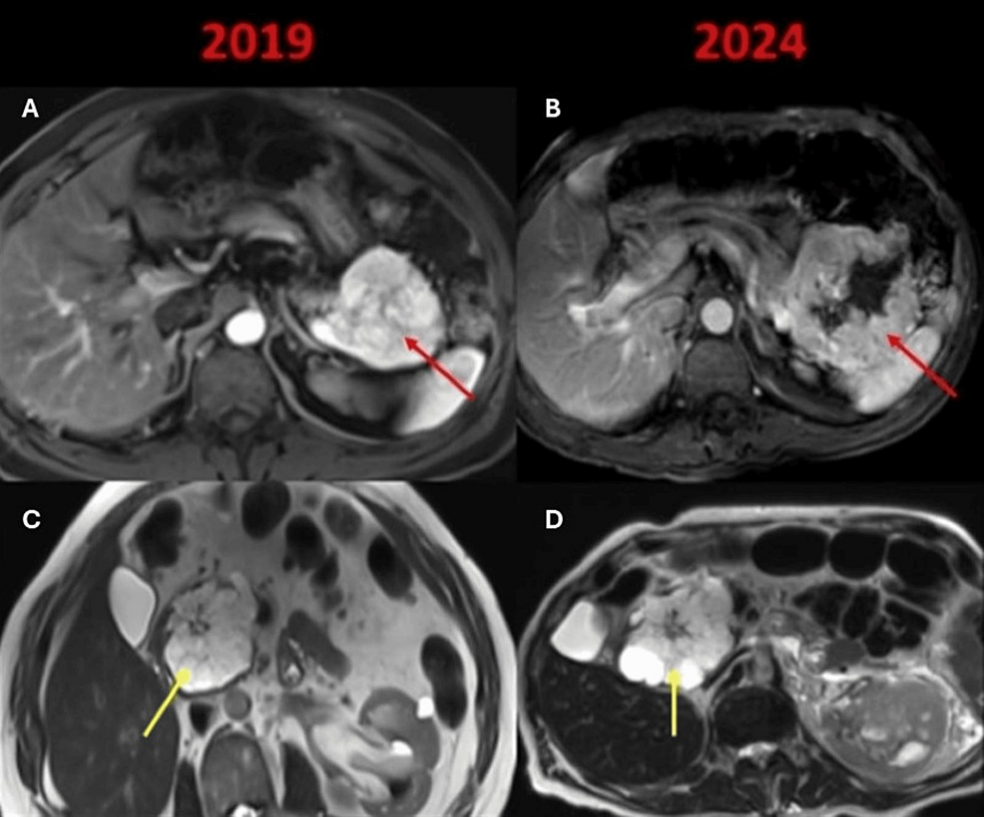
Comparative MRI between diagnosis and five years later. Comparison of imaging follow-up is made five years later. A and B: The neuroendocrine tumor in the tail of the pancreas presents an increase in size at the expense of the cystic degeneration that these tumors can suffer (red arrows). C and D: The lesion of the pancreatic head has no significant changes (yellow arrows), which confirms the benignity of the lesion (serous cystic tumor).
